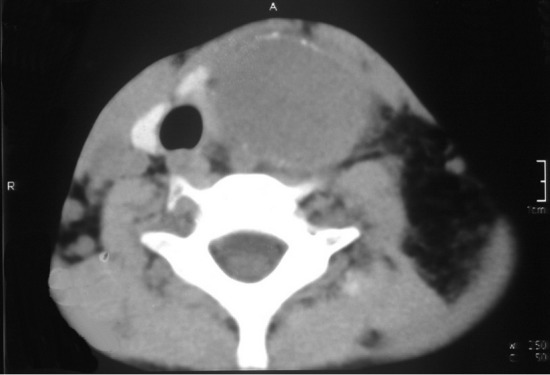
CT scan showing that the mass extends into the left thyroid gland. CT, computed tomography.
